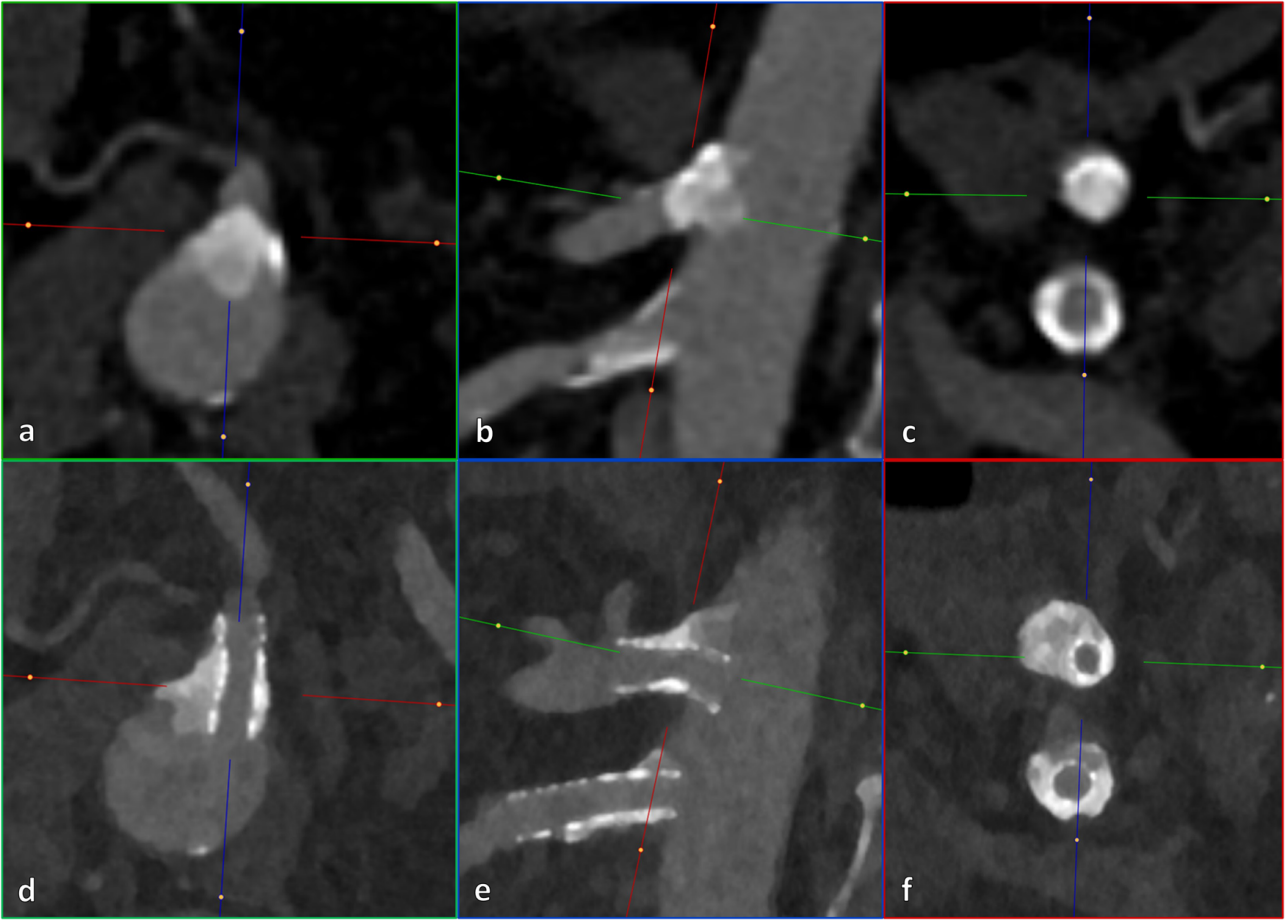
3D-reformatted CT angiography in paraaxial (green), parasagittal (blue), and paracoronal (red) views before and after IVL-assisted double-vessel bare-metal stent placement in a 64-year-old patient with chronic mesenteric ischaemia. The upper row (a–c) shows the preprocedural status of the superior mesenteric artery and coeliac artery: a calcified coeliac short-segment chronic total occlusion, and a relevant superior mesenteric artery stenosis due to a mixed atherosclerotic plaque with circumferential calcium and negative remodelling. The lower row (d–f) demonstrates postprocedural results with substantial lumen gain, favourable stent expansion, and only mild residual stenosis at the point of maximal calcification
